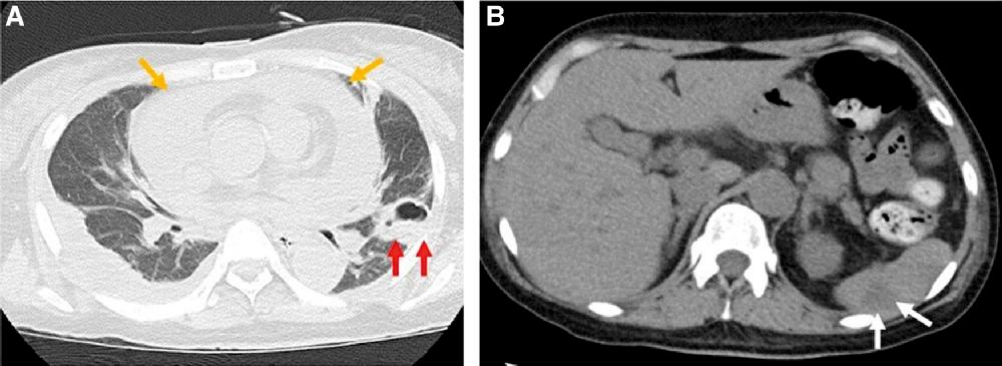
Computed tomography images of the chest at admission. Plain computed tomography of the chest shows bilateral pleural effusion, large pericardial effusion (orange arrows), splenic infarction (white arrows), and consolidation and cavity lesions in the upper-left lung zones (red arrows) (*A* and *B*).
